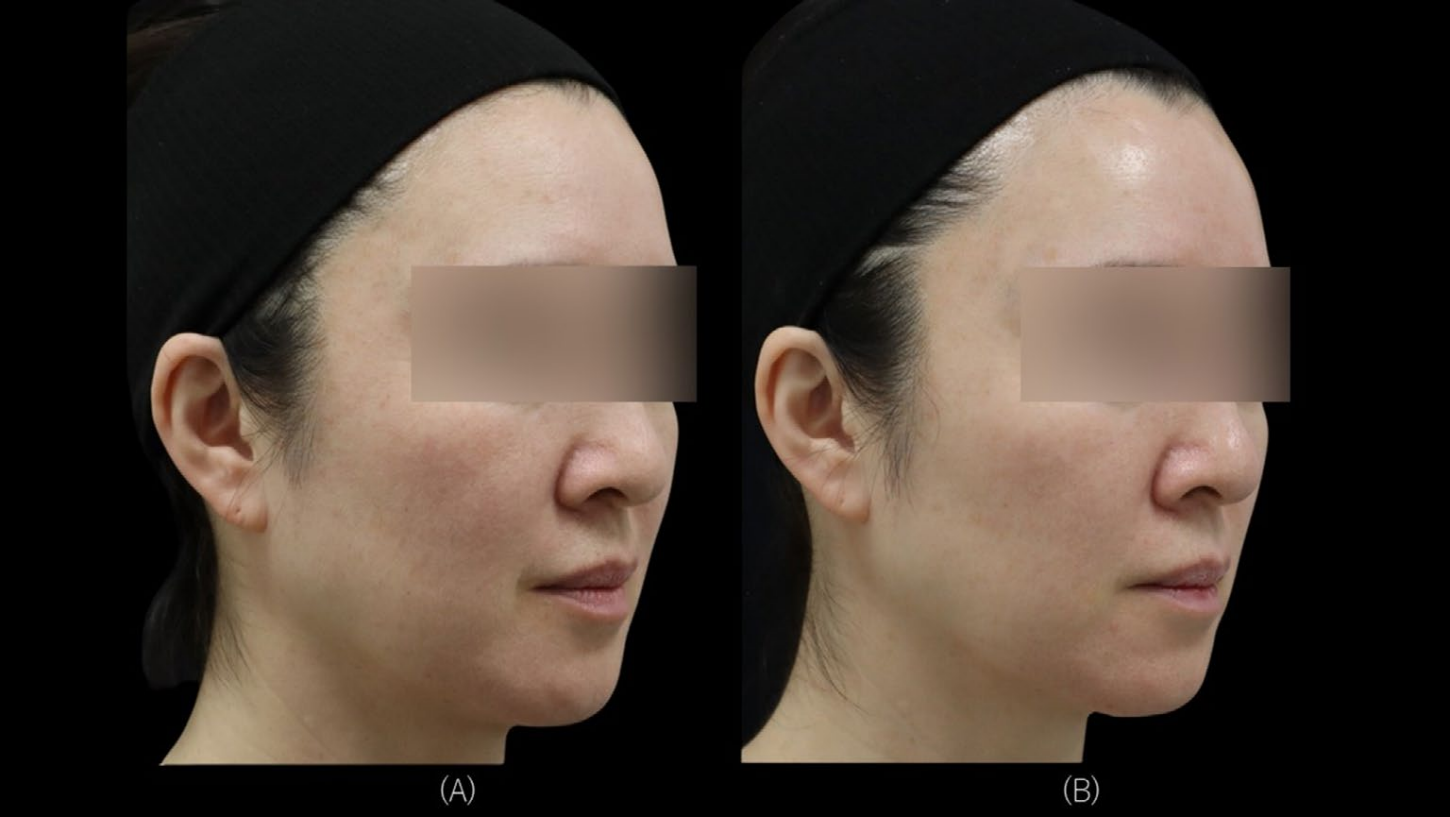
A 53‐year‐old female with Fitzpatrick Skin Type II. Treated with VYC‐12L and high intensity, high frequency parallel ultrasound beam. (A) Right side view at T0. (B) Right side view at T2.
